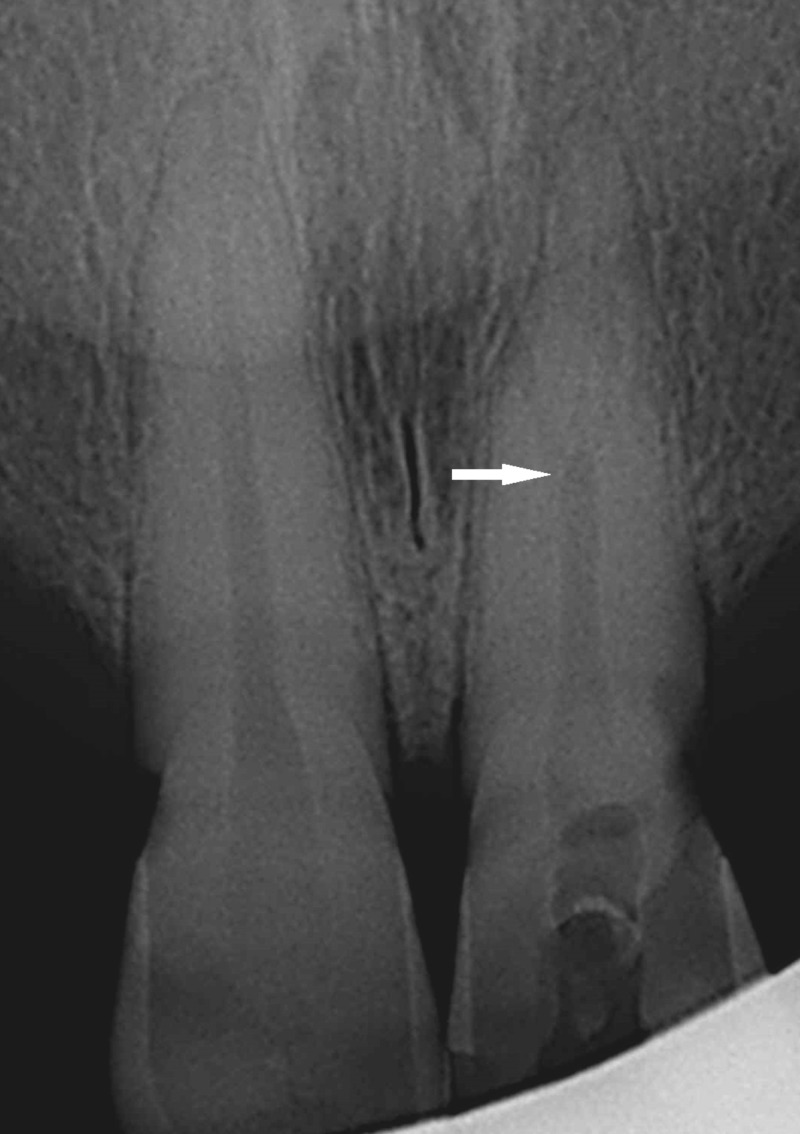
Preoperative periapical radiograph of tooth number 21 showing sudden break in root canal outline in the apical third (shown with arrow).
